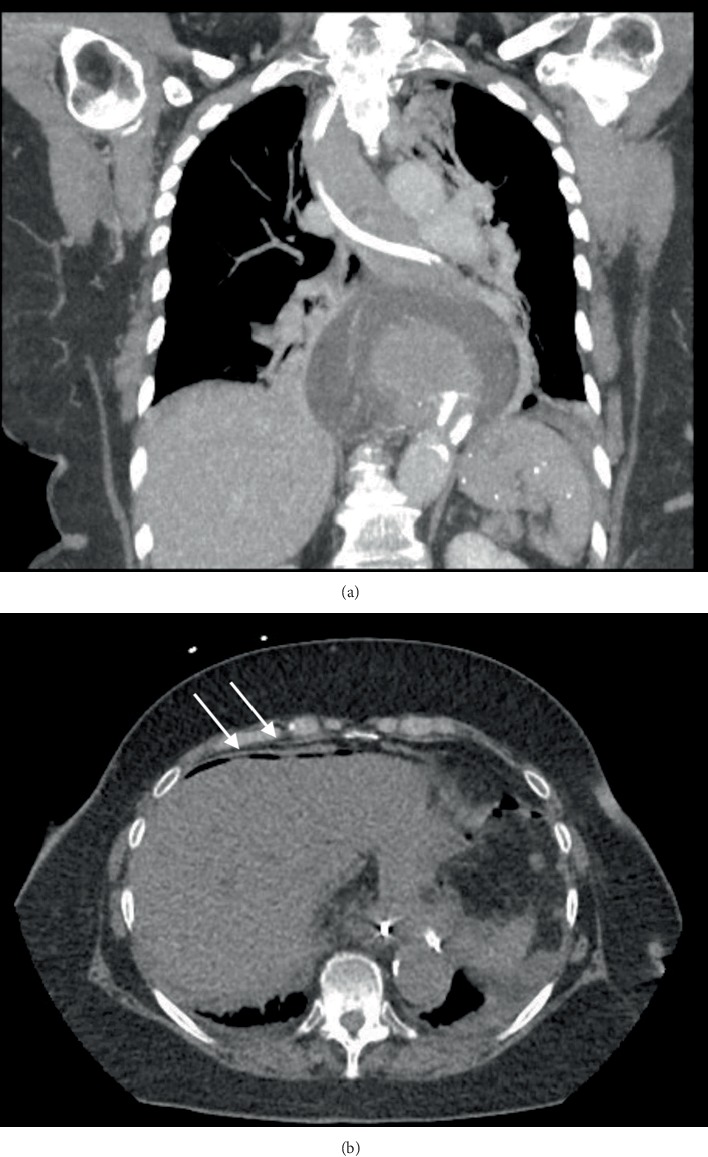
CT scan showing intra-abdominal and mediastinal free air and extraluminal contrast from the patient's gastric perforation. (a) Coronal view. (b) Axial view.
